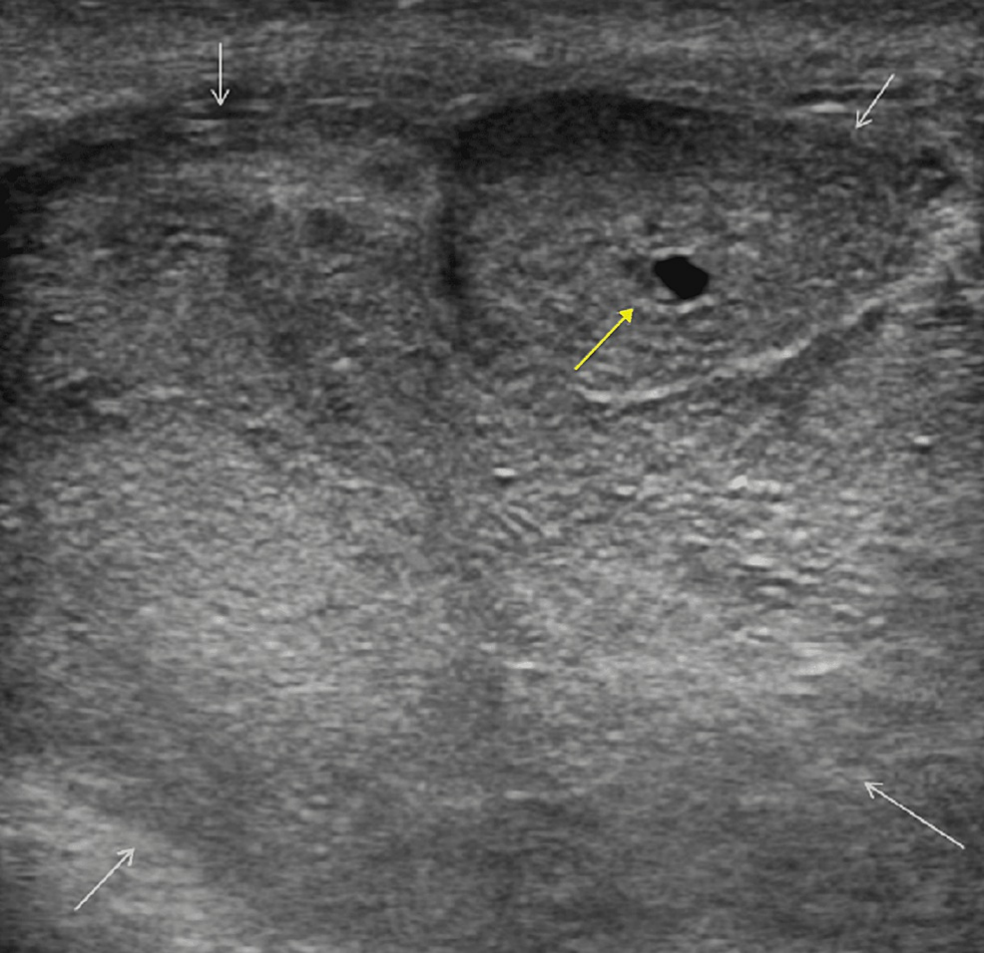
Ultrasound of right testis showing a mass lesion with heterogeneous echotexture including hypoechoic and hyperechoic areas (white arrow) and a small cystic area (yellow arrow).
